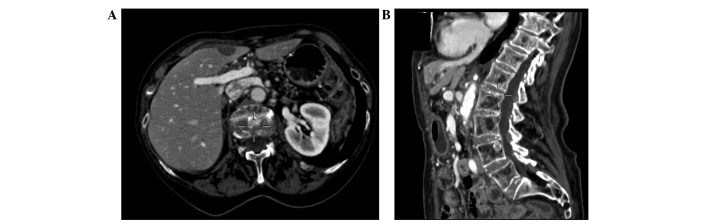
Stable liver and bone metastases during sunitinib treatment. (A) Liver metastasis in segment IV, showing hypodensity in the axial view of the computed tomography (CT)-scan. (B) Coronal view of the CT scan demonstrating multiple lytic vertebral metastases.
